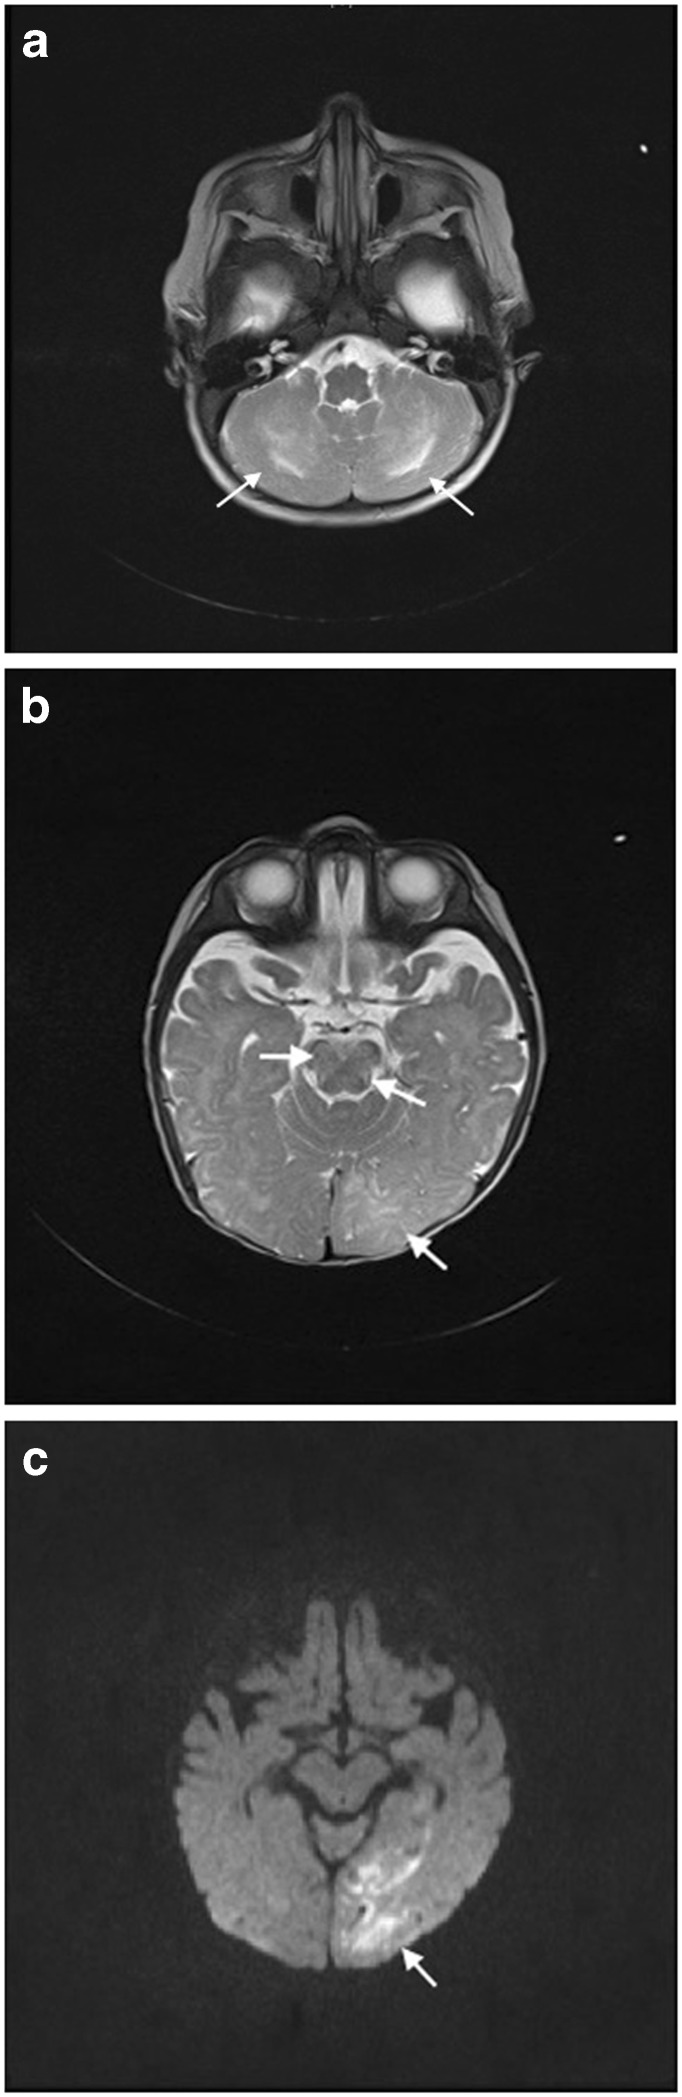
Neuroradiological MRI findings in HLH. a T2w image showing bilateral hyperintense lesions in the cerebellum. b T2w image with hyperintense signal and edema in the left posterior hemisphere and abnormalities in the brainstem. c Diffusion weighted imaging of the same region as in b with lesions imitating cerebral infarction.
